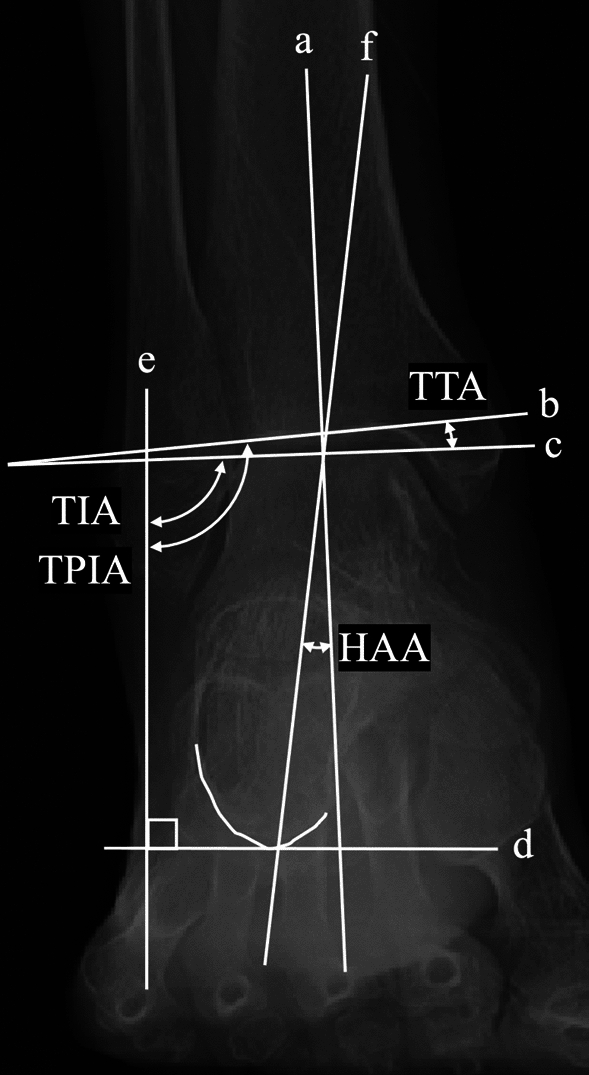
Radiographic measurements of ankle and subtalar joint alignment parameters on whole-leg anteroposterior weight-bearing radiographs. Line (a) represents the mechanical axis of the tibia, (b) the subchondral plate of the distal tibial articular surface, (c) a tangent to the talar dome, (d) a horizontal reference line parallel to the ground, (e) a line perpendicular to the ground, and (f) a line extending from the lowest point of the calcaneus to the center of the talar dome. The tibiotalar tilt angle (TTA) is defined as the angle between lines (b) and (c), the tibial plafond inclination angle (TPIA) is the angle between lines (b) and (e), the talar inclination angle (TIA) is the angle between lines (c) and (e), and the hindfoot alignment angle (HAA) is the angle between lines (a) and (f)
